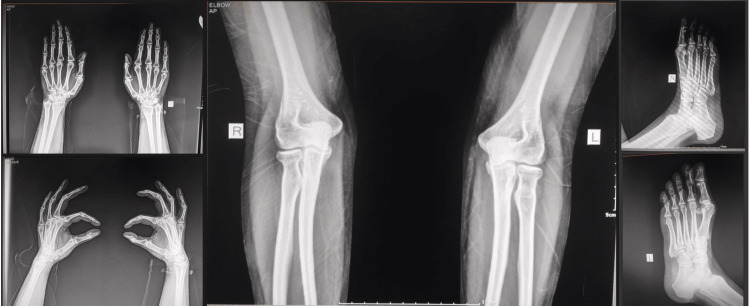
Plain X-ray of the right and left hand AP and lateral view. Plain X-ray of the right and left elbow showing the distal part of the humerus and proximal part of the radius and ulna AP view. Plain X-ray of the right and left foot with the distal end of the tibia and fibula in oblique view AP view: anteroposterior view; R: right; L: left
